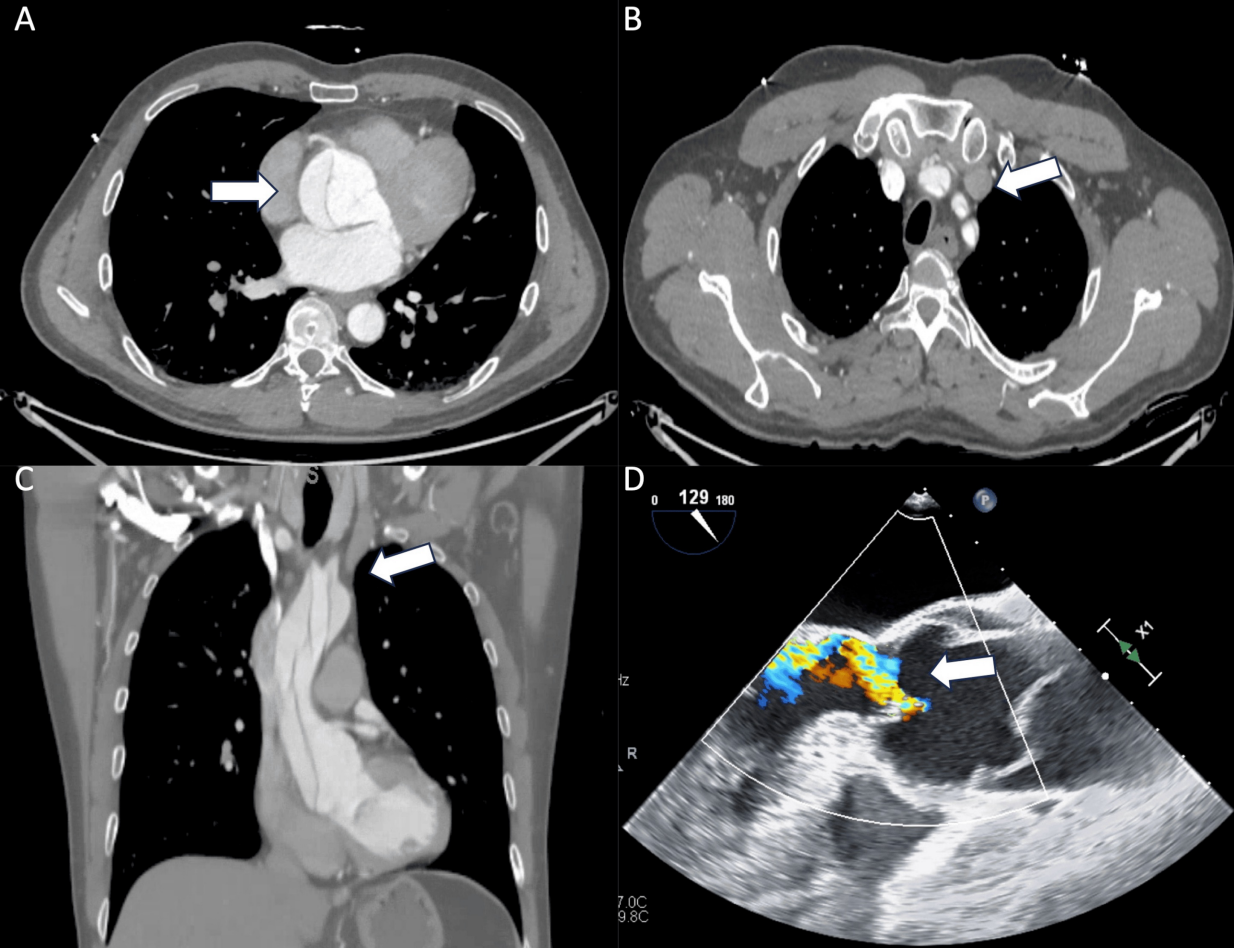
Preoperative chest computed tomography with contrast (A) Aortic dissection arising from the aortic root (white arrow). The aortic root is 6.2 x 5.0 cm in diameter (B, C). Aortic dissection extended into the innominate artery, the left carotid artery, and the left subclavian artery (white arrow). (D) Transesophageal echocardiogram: moderate aortic insufficiency (white arrow)
